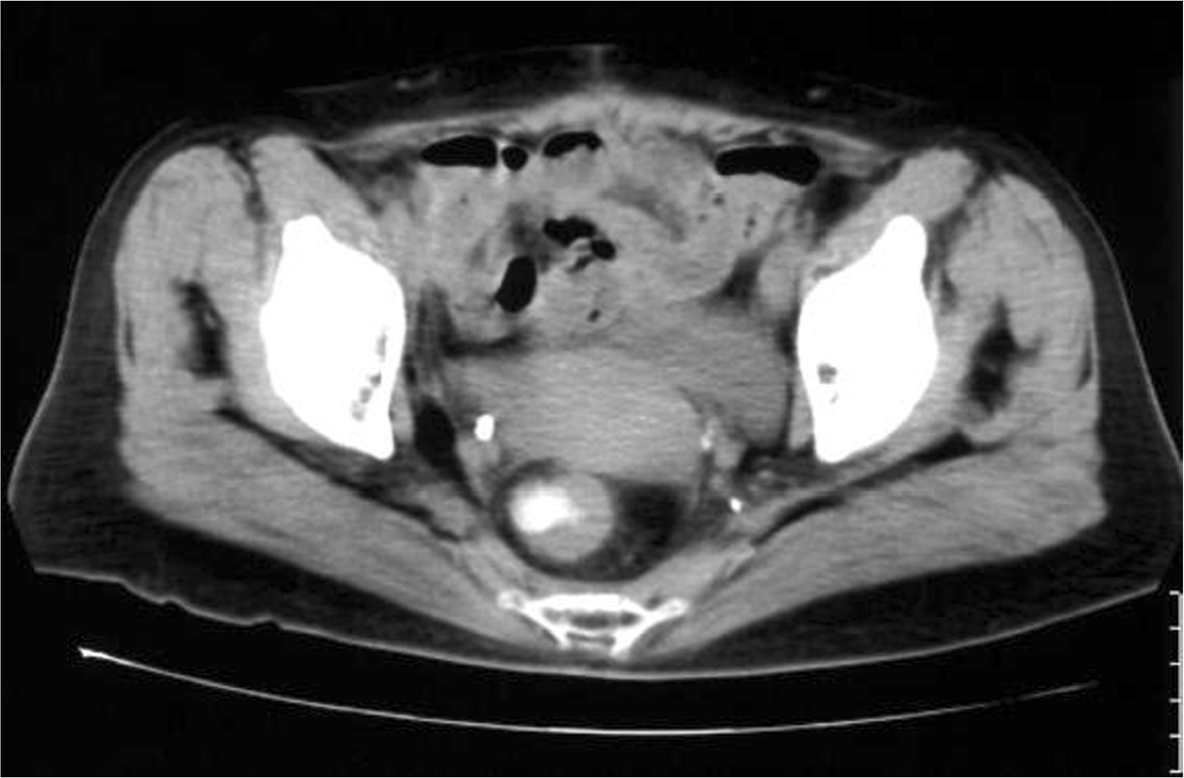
An abdominal computed tomography image showing rectal stenosis with thickening of the rectosigmoid wall.
